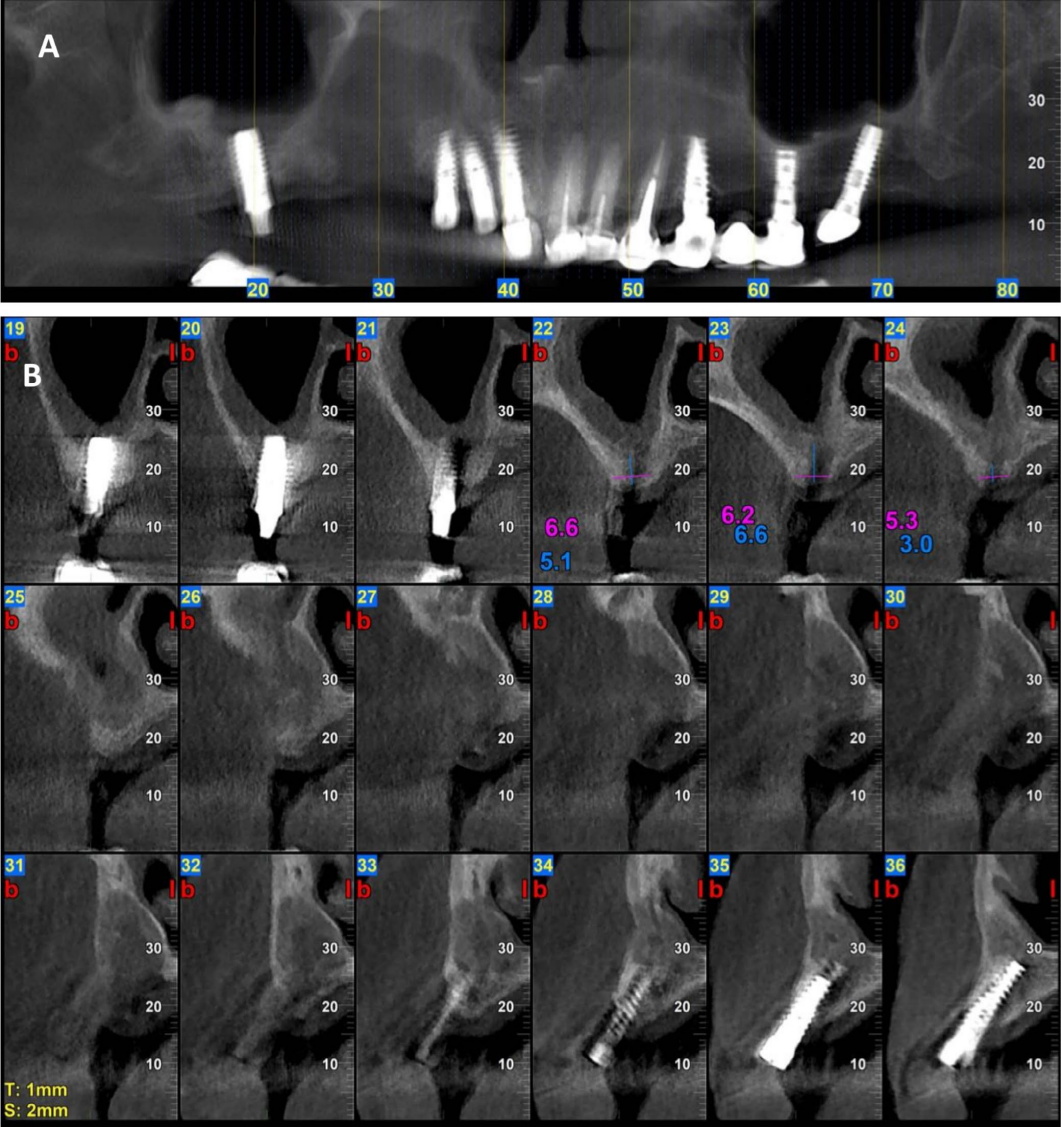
CBCT 4 months follow‐up CBCT post marginal maxillectomy and GBR—Case 3. (A) Panoramic reconstruction from CBCT 4 months after dental implant extraction, marginal maxillectomy of the osteolytic lesion and GBR. (B) Axial CBCT view demonstrating the maxillary defect.
